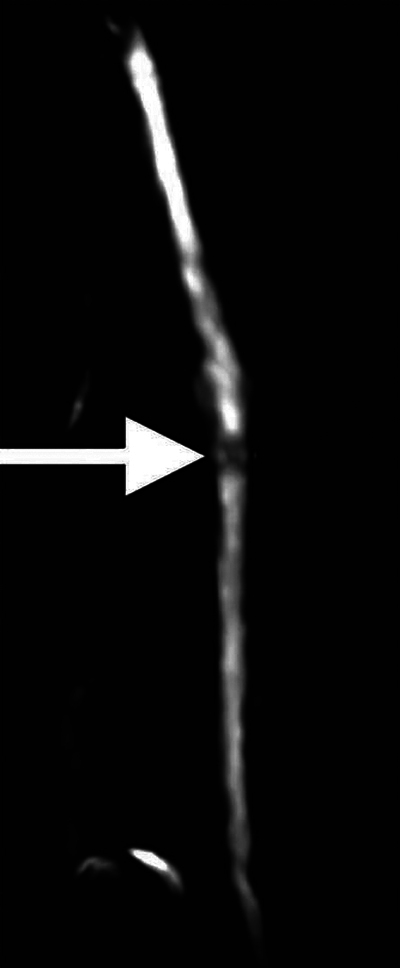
MRI with neurographic 3D STIR sequences shows hyperintense diffuse thickening of the radial nerve with a focal area of narrowing (arrow).
